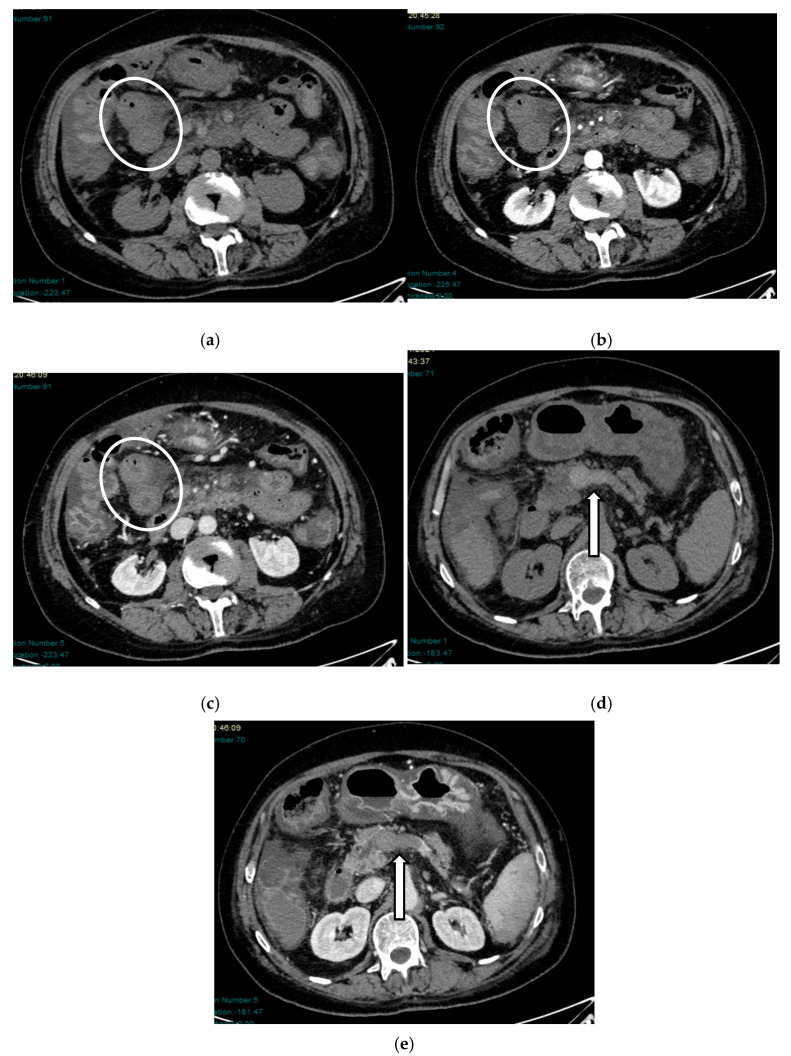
Thrombotic AMVI in a 60-year-old man: axial pre- and post-contrast scans show some jejunal loops with thickened and shaded walls and low enhancement (circles) (a–c). A marked mesenteric thickening caused by hemorrhagic infarction can also be appreciated. Extensive thrombosis of the portal vein appears hyperdense in an unenhanced CT scan (arrows) (d,e).
